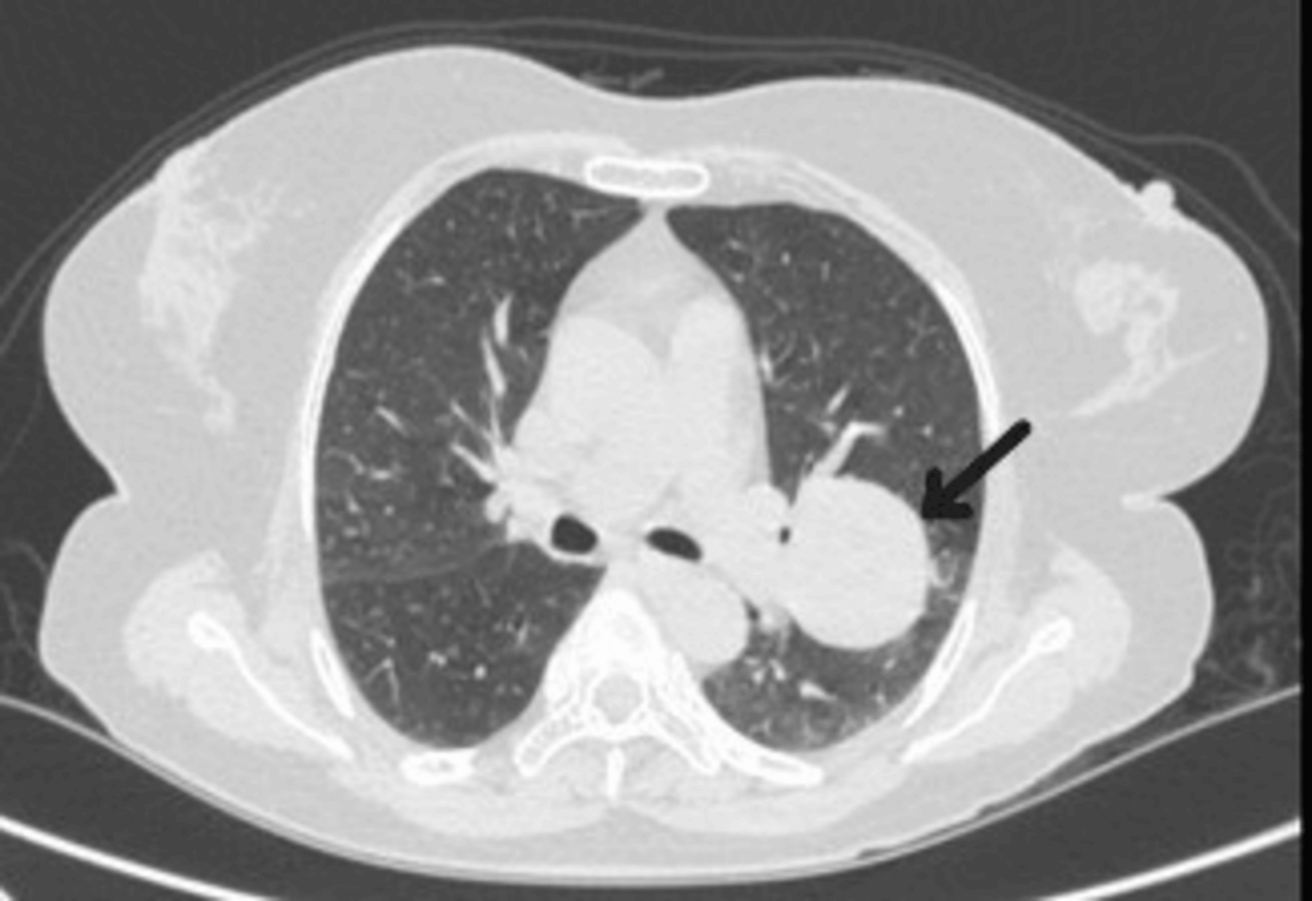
Contrast-enhanced computed tomography of the thorax axial section (lung window) showing a well-defined smoothly marginated heterogeneously enhancing soft tissue density lesion in the left upper lobe
